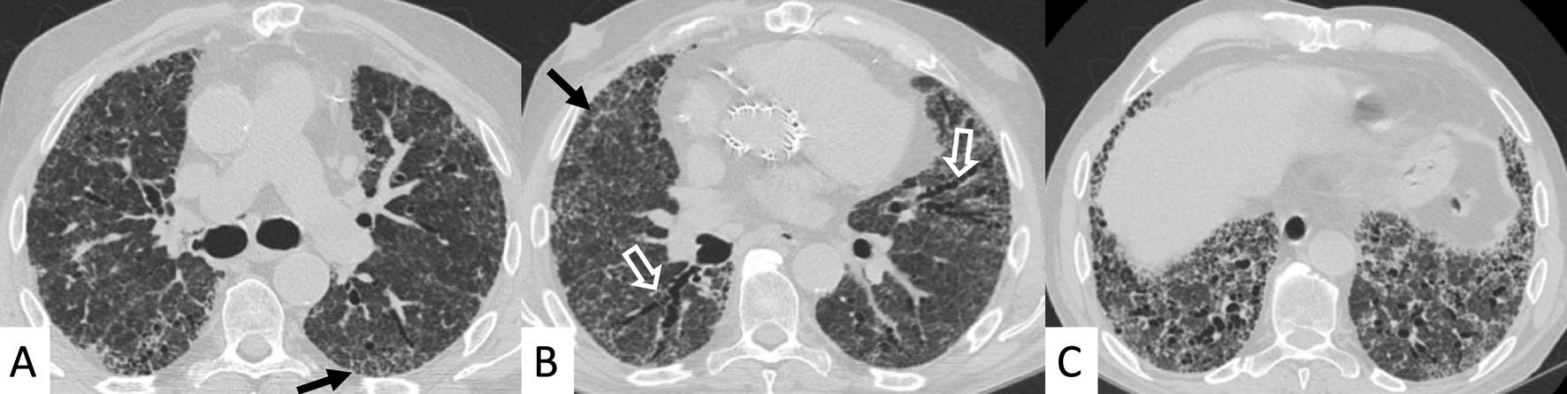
A 70-year-old man with MCTD, positive for ANCA antibodies. HRCT images show reticulations (black arrows in A, B) and bronchiectasis, with irregular course through the lung (white empty arrows), and cystic appearance, clearly recognizable in the basal regions in C
